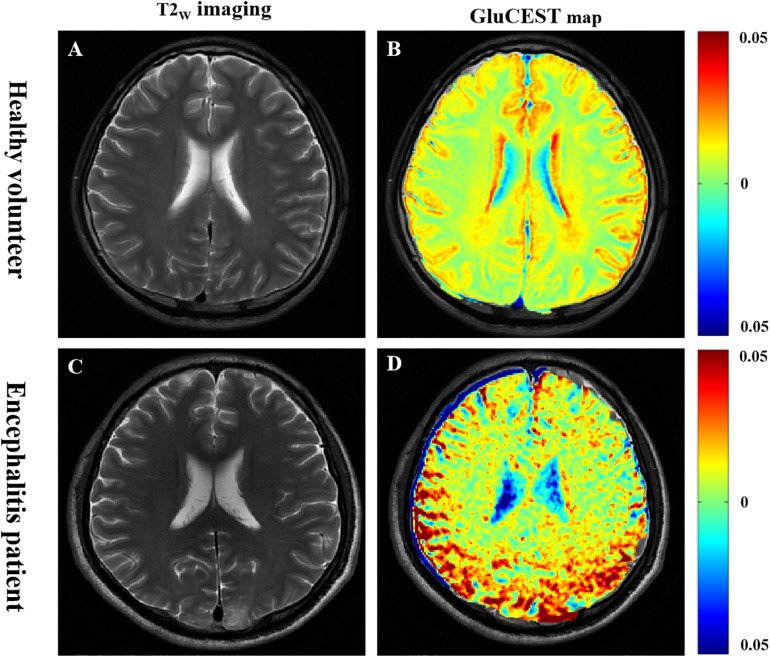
Glutamate chemical exchange saturation transfer imaging was feasible in human brain using the 3.0 T MR system. (A,B) In a healthy volunteer, GluCEST magnetic resonance image (MRI) could clearly distinguish white matter and gray matter, similar to T2_W_ imaging, and the gray matter signal intensity of GluCEST was higher than that of white matter. (C,D) In a patient with encephalitis, GluCEST imaging also clearly showed the left parietal cortex and subcortex lesion.
